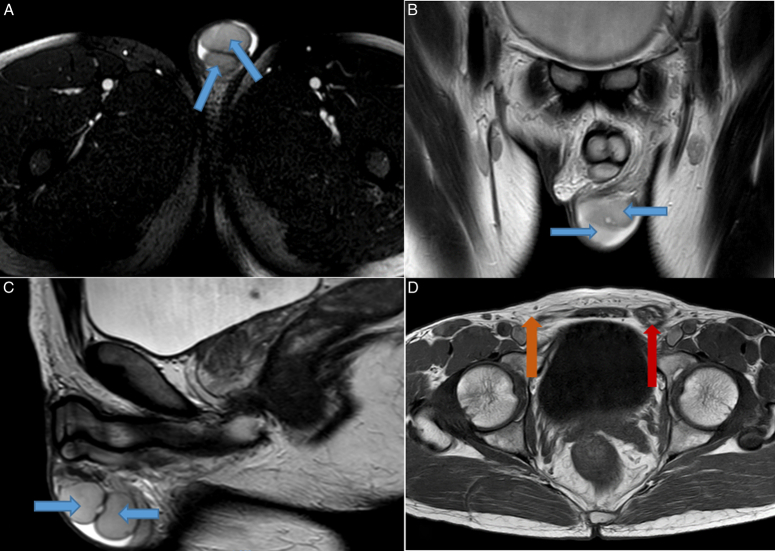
MRI images of scrotum and pelvis. (A) Axial T2W(SPIR) image shows two testes in the left hemiscrotum (blue arrows) and an empty right scrotal sac. (B, C) Coronal T2W and sagittal T2W images, respectively, show two testes in the left hemiscrotum (blue arrows). (D) Axial T2W image reveals fused left-sided vas deferens (red arrow), which divide to approach both testes and the absence of right-sided vas deferens (orange arrow).
